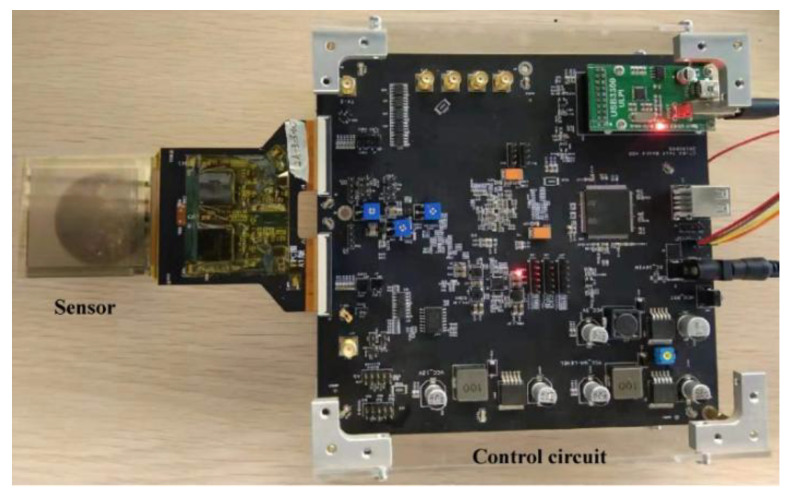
Prototype of the proposed fourth-order LCLC converter to drive an ultrasonic fingerprint sensor.
